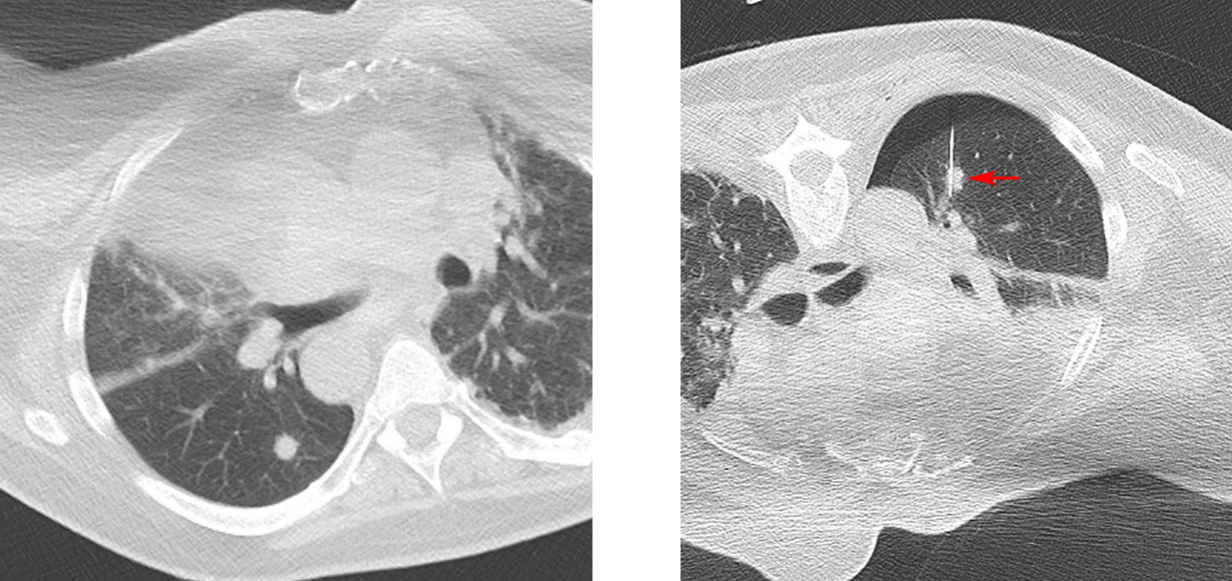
Example of likelihood of adequacy score 2 (equivocal). (A) Chest CT showing right lung nodule that is small and in location (behind rib) requiring angled approach (B) Arrow points to needle in right lung nodule. Small pneumothorax is noted.
